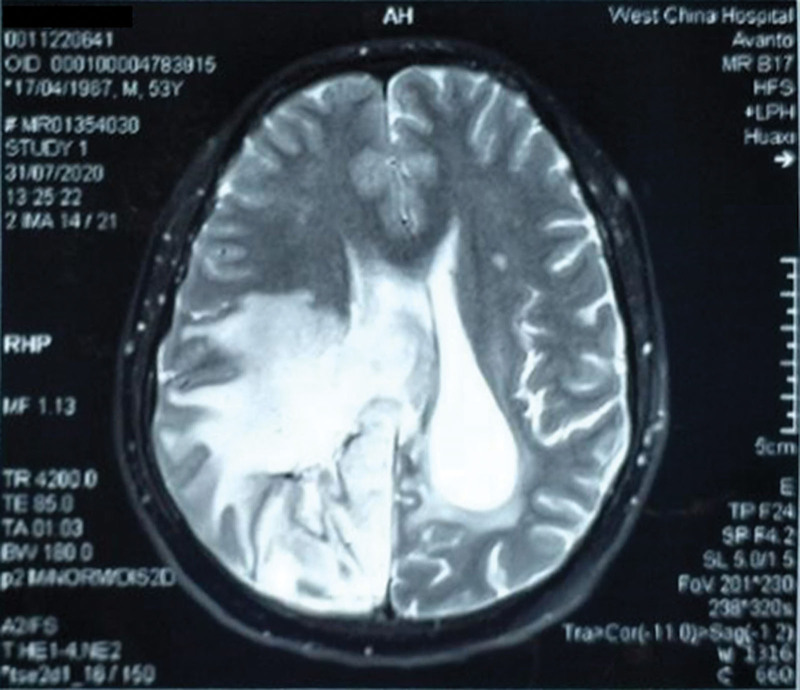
In July 2020, MRI indicated the size of the target lesion at 5.3 × 4.6 cm. MRI = magnetic resonance imaging.
